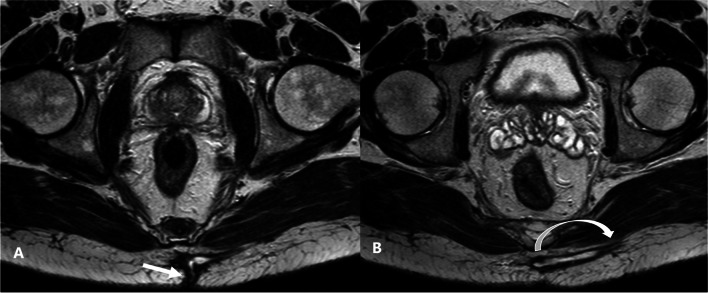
Axial T2-weighted image of the natal cleft of a 43-year-old male patient complaining of recurrent natal cleft discharge: (A) midline skin opening (arrow), (B) left natal cleft lateral branching to the subcutaneous gluteal region (curved arrow)
